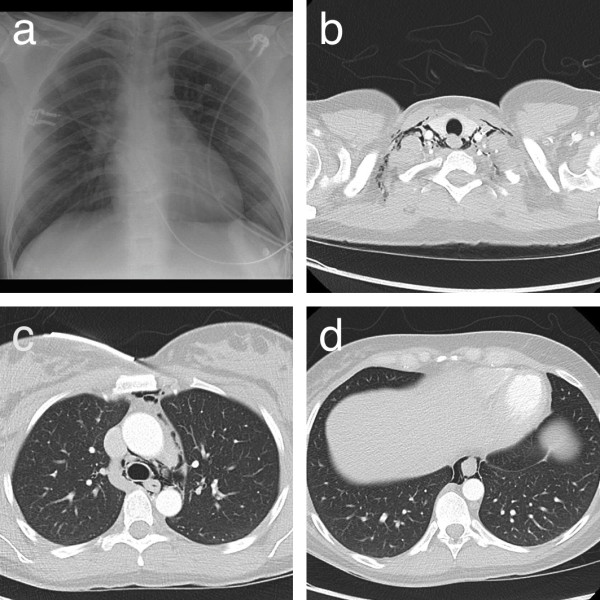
X-ray and chest computed tomography at initial presentation. a shows the chest X-ray of the patient that reveals a thin stripe of increased transparency along the left side of her trachea. b to d show slices of the chest computed tomography that reveal cervical and mediastinal emphysema.
